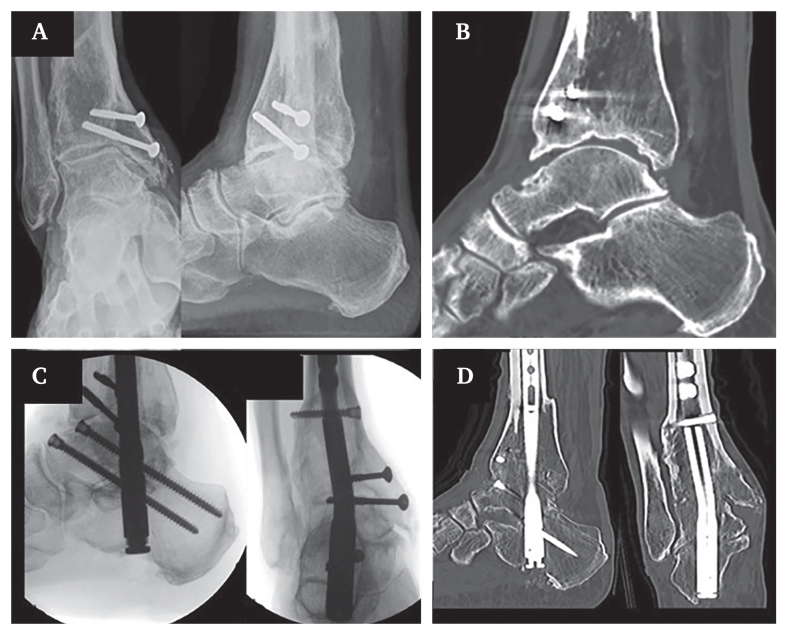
(A) Pre-operative radiographic and (B) computed tomographic imaging of the right hindfoot documenting end stage, multiplanar varus osteoarthritis of the ankle and the subtalar joint. (C) Intra-operative radiographic and (D) post-operative computer tomography imaging revealing good bony contact at the arthrodesis sides and an acceptable hindfoot alignment.
